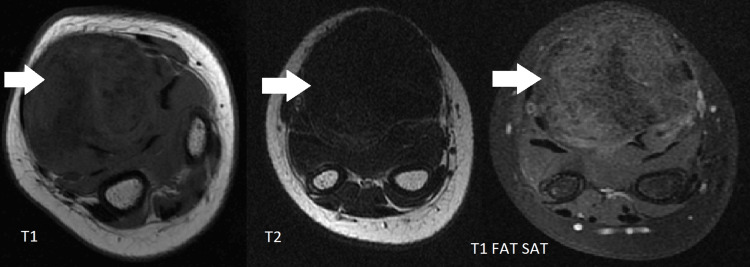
MRI of the wrist in axial T1, T2, and post-contrast T1 fat-saturated sequences demonstrating a roughly ovoid lesion on the anterior aspect of the wrist, with macrolobulated margins. The lesion is isointense on T1 with internal hypointense areas, markedly hypointense on T2, and shows mild, progressive enhancement following contrast administration, suggestive of a fibrous nature.
